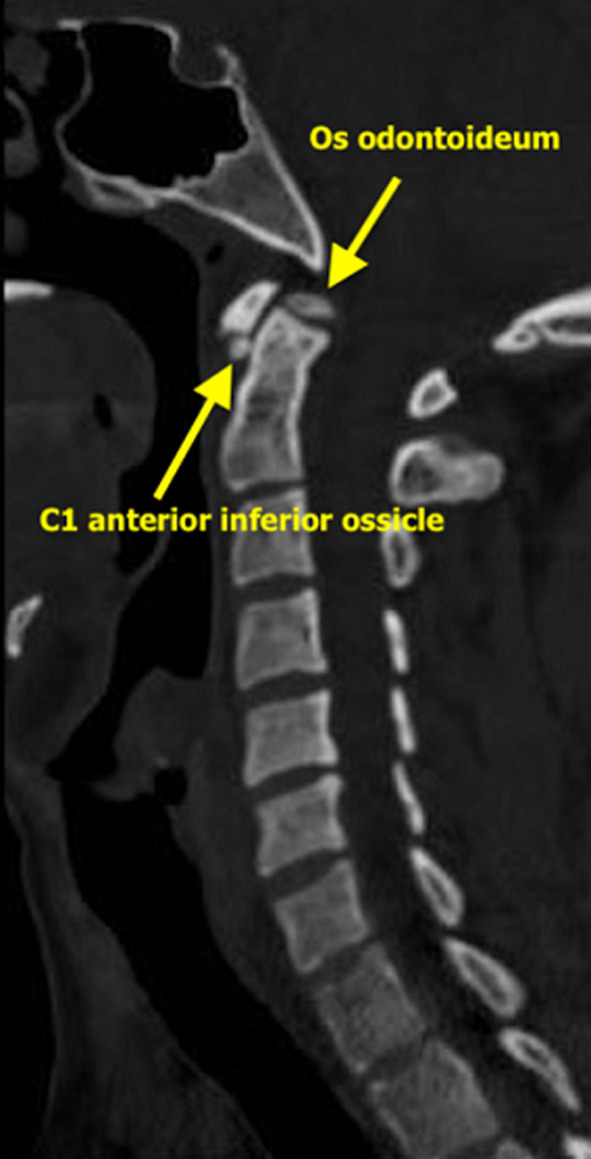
sagittal view of neck CT scan is showing dual cervical (C1-C2) ossicles
